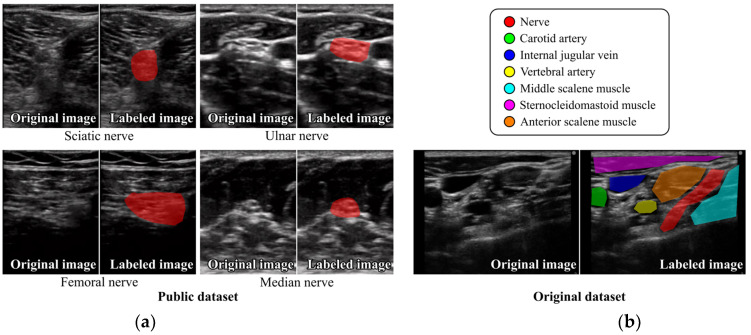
Examples of ultrasound images and their corresponding labeled images in (a) Public dataset and (b) Original dataset.
